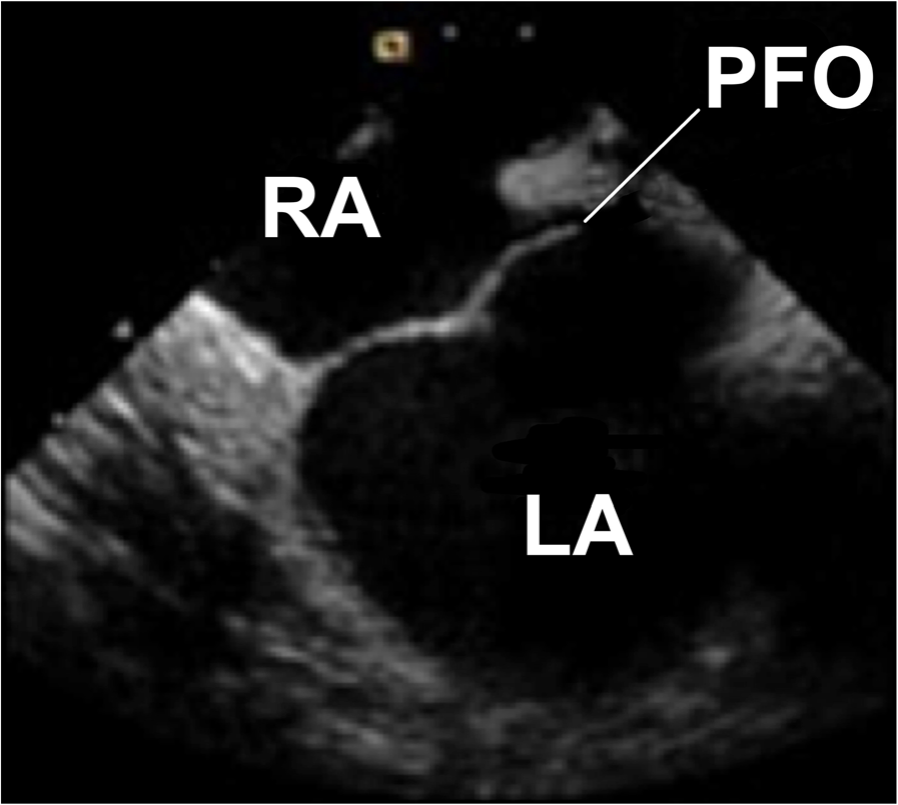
Intra-cardiac echocardiogram. Showing the patent foramen ovale, in an adult and in real time, acting as a flap valve between the right atrium (*RA*) and left atrium (*LA*) [6].
